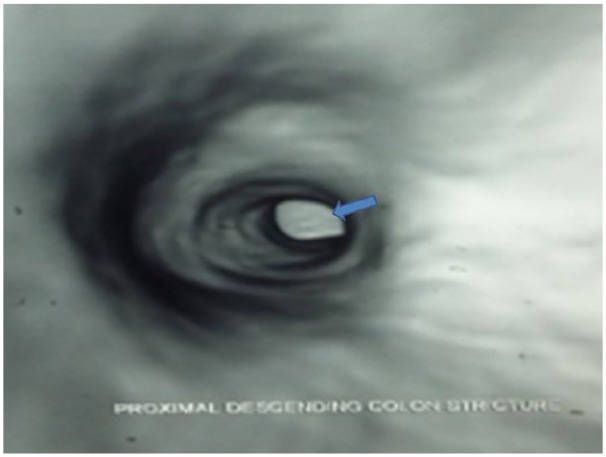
Computed tomography colonoscopy revealed descending colon stricture of approximately 6 cm in length.
